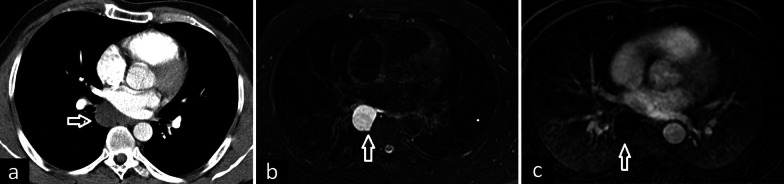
CT and MRI features of bronchogenic cyst. Low-density mass behind the left atrium is seen on contrast-enhanced axial CT image (a, arrow). Axial T2-weighted MR sequence (b) demonstrates uniform high signal intensity similar to cerebrospinal fluid and contrast-enhanced T1-weighted image (c) demonstrates the absence of contrast enhancement within the cyst (arrows)
